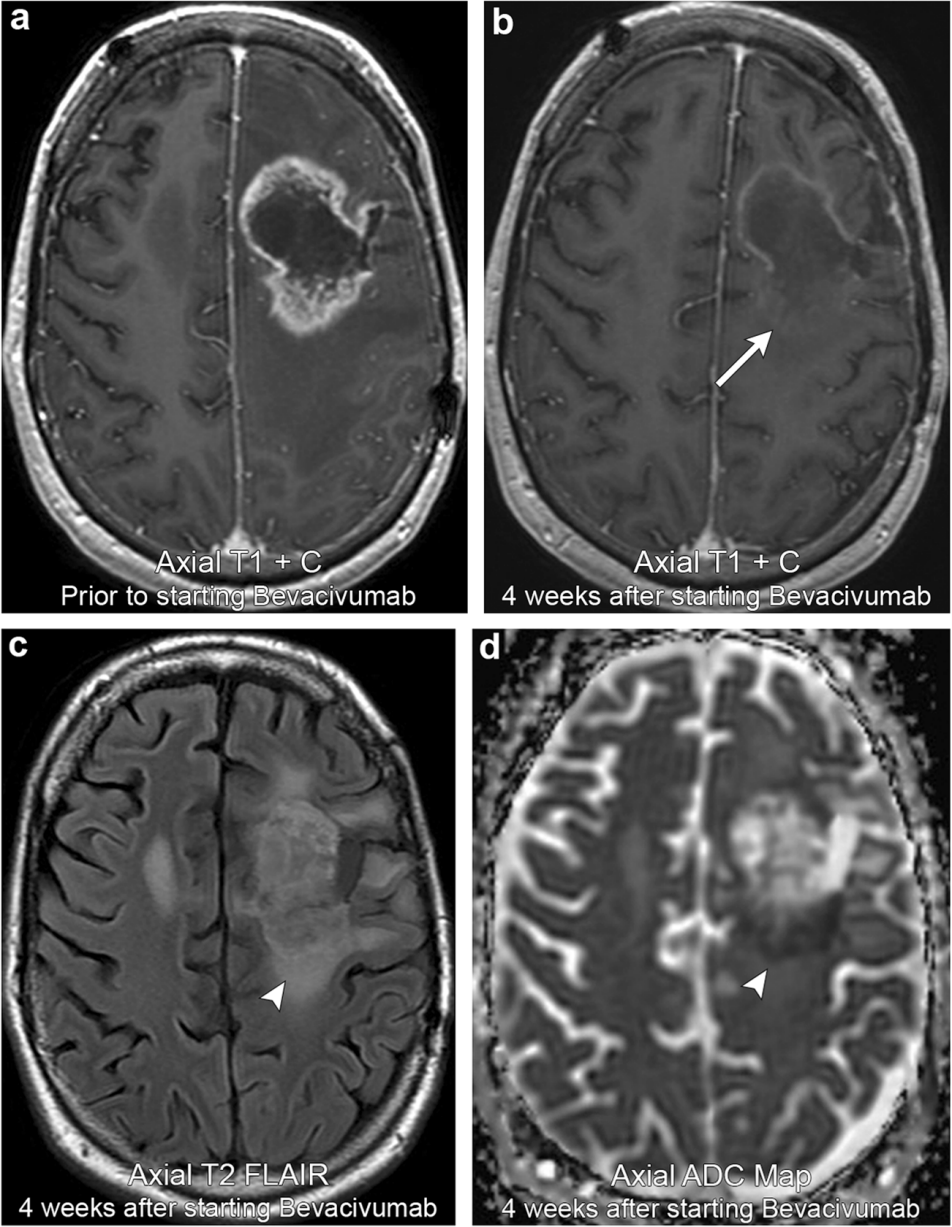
Pseudoresponse. A 38-year-old male with left frontal astrocytoma. Initial imaging demonstrates a peripherally enhancing lesion in the left frontal lobe. Follow-up imaging 4 weeks after starting bevacivumab demonstrates a marked interval decrease in enhancement (arrow). However, there is persistent T2 FLAIR hyperintensity and low ADC signal (arrowheads). Serial imaging afterwards (not shown) demonstrated progression in T2 FLAIR hyperintensity and mass effect, confirming pseudoresponse
